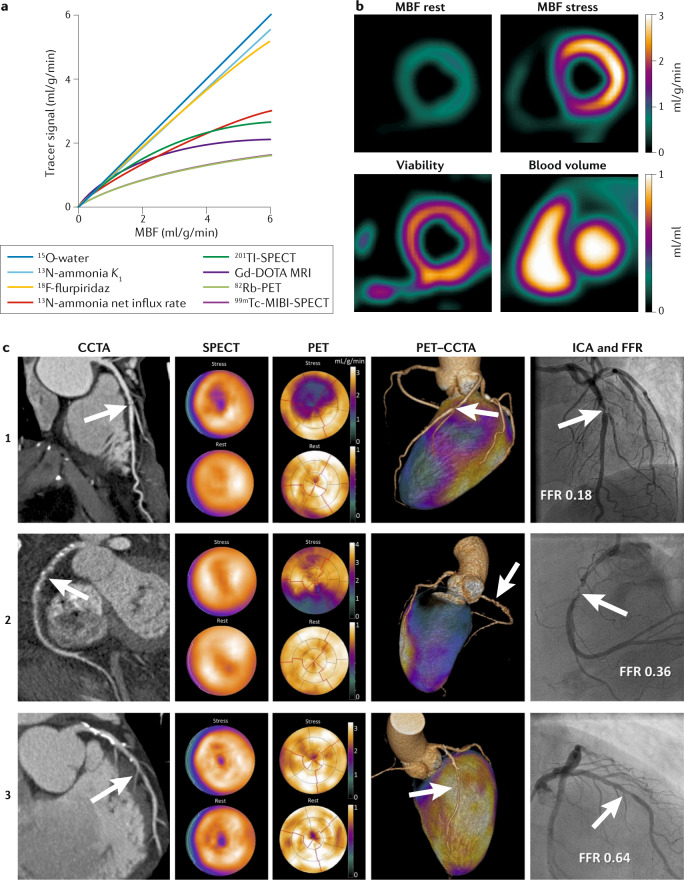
PET for quantification of myocardial ischaemia. a | Tracer signal versus myocardial blood flow (MBF), showing a constant 100% extraction for ^15^O-water, an almost 100% extraction for ^13^N-ammonia *K*_1_ and ^18^F-flurpiridaz, and a substantial roll-off phenomenon, resulting in underestimation of MBF that increases with MBF, for ^13^N-ammonia (when quantified using its net influx rate), ^201^Tl-SPECT, Gd-DOTA dynamic contrast-enhanced (DCE) MRI, ^82^Rb and ^99m^Tc-MIBI-SPECT^66,189–192^. Note that the ^82^Rb and ^99m^Tc curves overlap. b | MBF images at rest and during stress, myocardial viability and blood volume from a single rest–stress ^15^O-water protocol. This protocol can be used to assess ventricular volumes and ejection fraction. c | Example images from the PACIFIC trial showing coronary CT angiography (CCTA), SPECT, PET and invasive coronary angiography (ICA) images, with corresponding fractional flow reserve (FFR), in three patients (numbered 1–3) with coronary artery stenosis (arrows).
